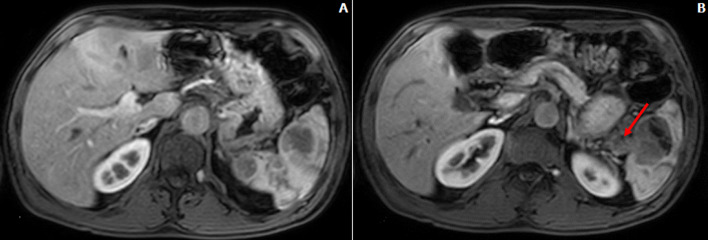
(A,B) abdominal MRI findings
